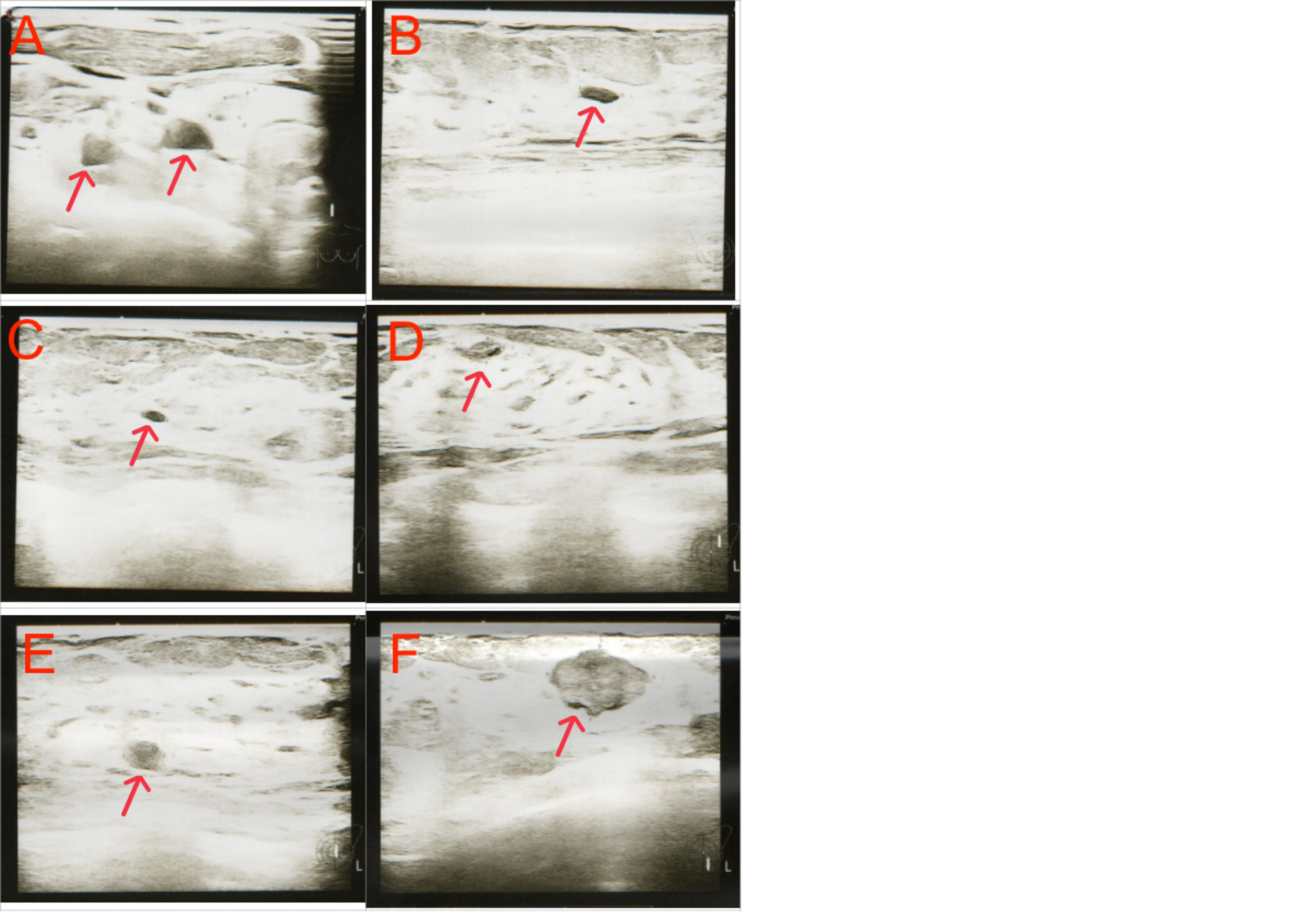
Breast ultrasound examination on March 17, 2021 A: Cysts measuring 7.3 mm and 4.1 mm in diameter; B: Cyst measuring 7 mm in diameter; C: Cyst measuring 4.3 mm in diameter; D:  Hypoechoic transformation measuring 8×4 mm, suggestive of a milk duct filled with dense content; E: Cyst measuring 6.8 mm in diameter; F: Hypoechoic lesion measuring 18×13×13 mm with increased vascular flow.
